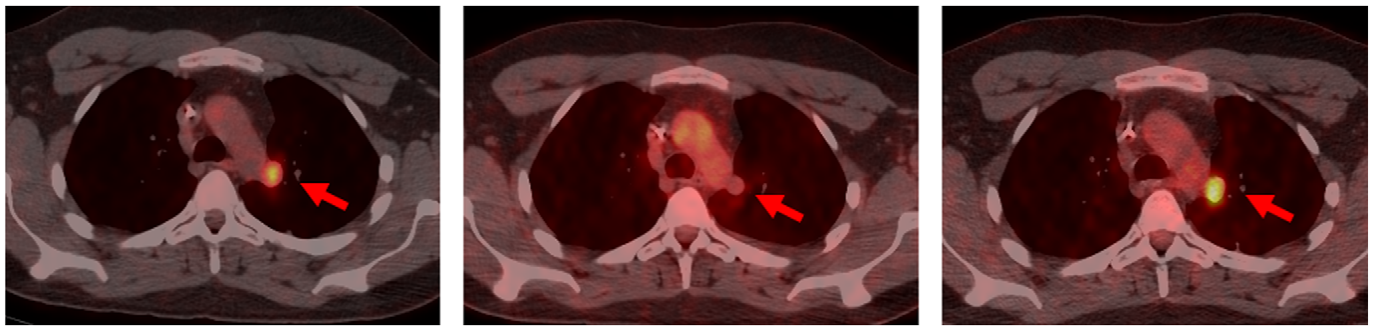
Imaging Responses in Ewing's sarcoma patient 2. FDG PET /CT in patient 2 with Ewing's sarcoma response to IMCA12+Temsirolimus combination and then emergence of resistance. Left panel shows pre-treatment FDG PET/CT scan of the thorax showing metastatic Ewing's sarcoma in the lung. Middle panel shows FDG PET/CT response after 8 weeks of treatment. Right panel shows re-emergence of resistance 16 weeks after IGF1R antibody+ mTOR (IMCA12+ Temsirolimus) therapy.
